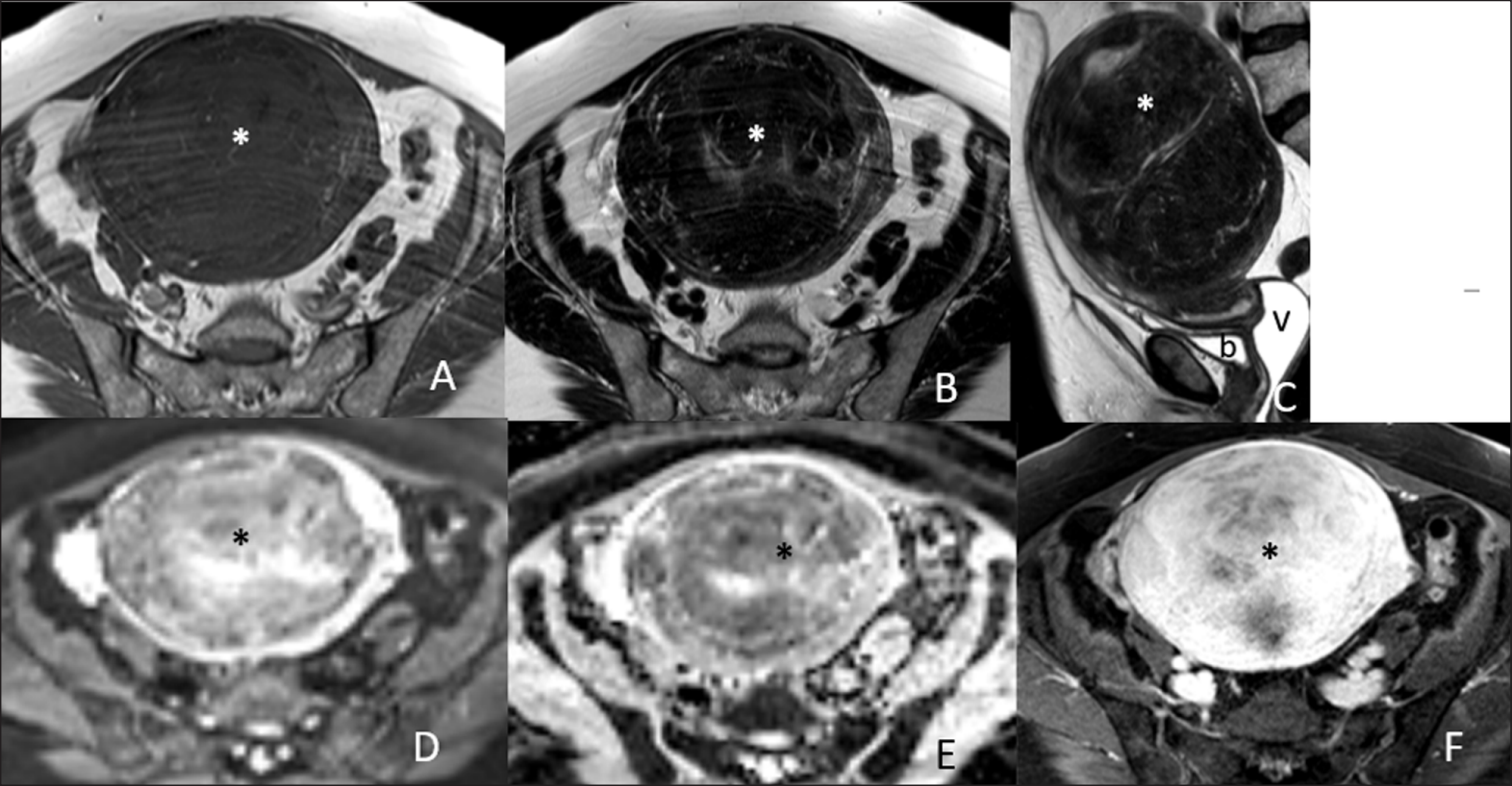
60-year-old woman with LM. (A) Axial T1WI, (B) axial T2WI, (C) axial diffusion and (D) ADC maps MRI show a large, heterogeneously T1 and T2 low signal intensity LM (asterisk).
